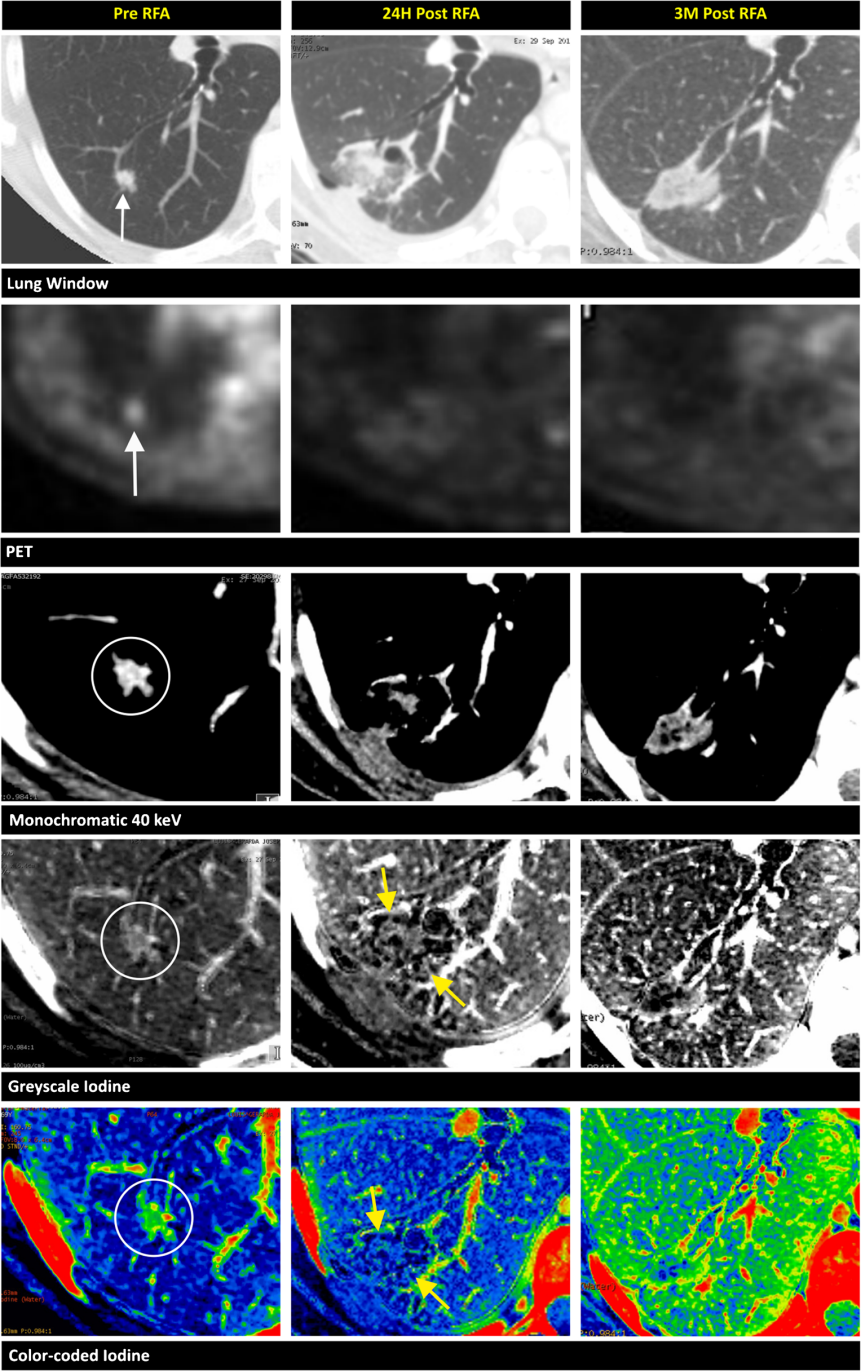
Pulmonary metastases. A 69-year-old female, with a history of breast cancer diagnosed 9 years prior, shows a hypermetabolic nodule in the inferior lobe of the right lung on PET/CT. A biopsy reveals a metastasis of a ductal mammary carcinoma. An RF ablation is performed. Imaging of the lesion 1 day before (first column), 24 h after (second column), and 3 months after (third column) complete RF ablation is performed. Before ablation, an 8-mm well-circumscribed nodule is depicted on the synthesised monochromatic 70-keV lung window, FDG-avid on the PET scan (*white arrows*). Internal vascularisation is clearly observable on the contrast-enhanced, synthesised monochromatic 40-keV, greyscale- and colour-coded iodine images (*circles*). The changes found 24 h post ablation are a ground-glass appearance (*yellow arrowheads*) around the lesion and the vanishing of the previously seen iodine uptake in the lesion. The post-ablative avascular zone surrounding the necrotic lesion can be delineated on the iodine images as darker areas (*yellow arrows*). On the lung window images after 3 months, the treated area has transformed into a homogeneous, well-delineated area of high attenuation. PET/CT images reveal no activity after the RF ablation. The greyscale- and colour-coded iodine images show a reduction in size of the avascular region
